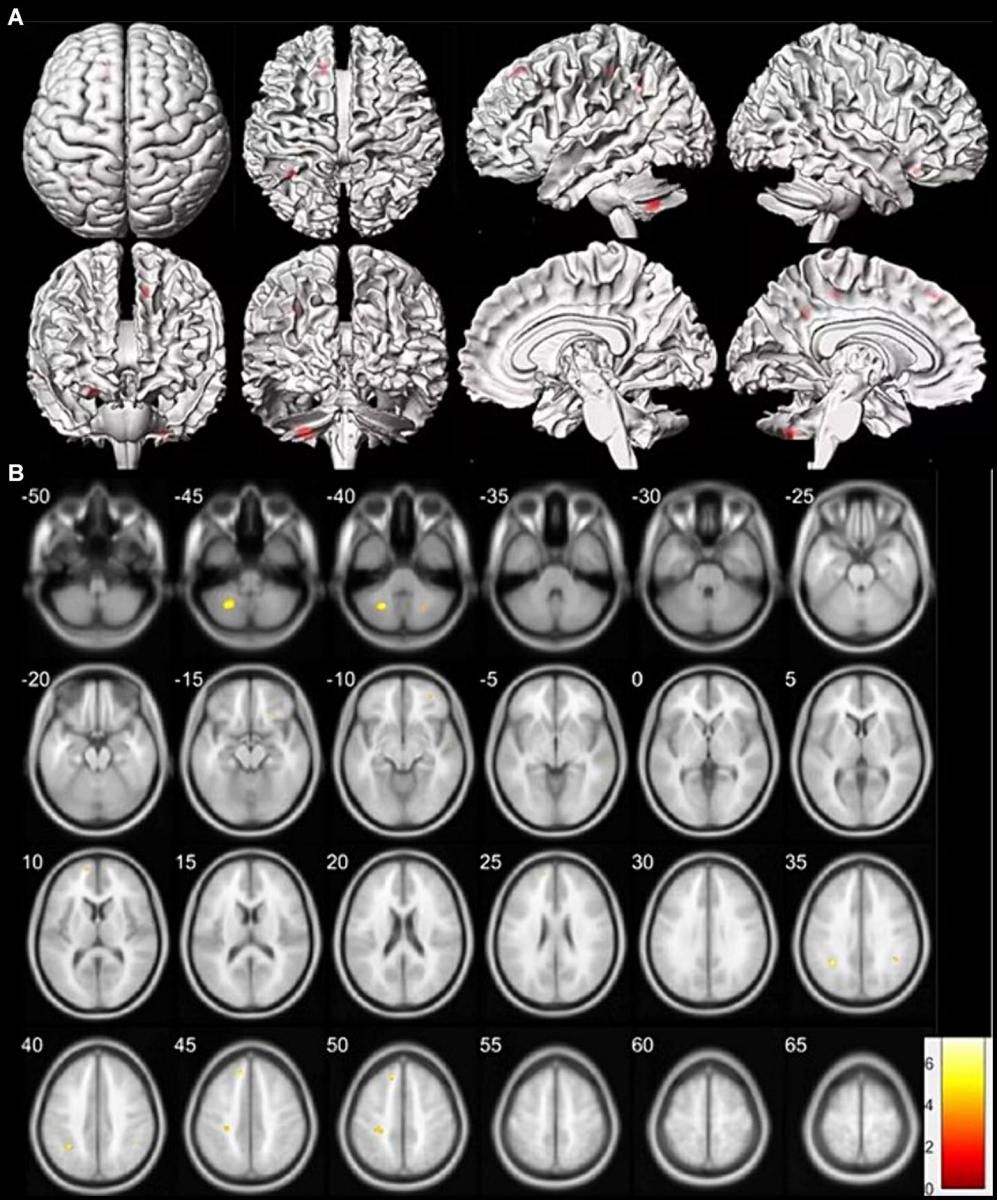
Clusters of synaptic change in SSNHL patients with ear fullness versus healthy controls (HCs). (A) The clusters of synaptic changes are shown on a three-dimensional brain template. The region of synaptic change displayed in red. (B) The clusters of synaptic changes are shown on a magnetic resonance imaging template. Low intake displayed in cool colors. Data were analysed at a height threshold of *p* < 0.001 and were cluster-level corrected for familywise error at *p* < 0.05.
